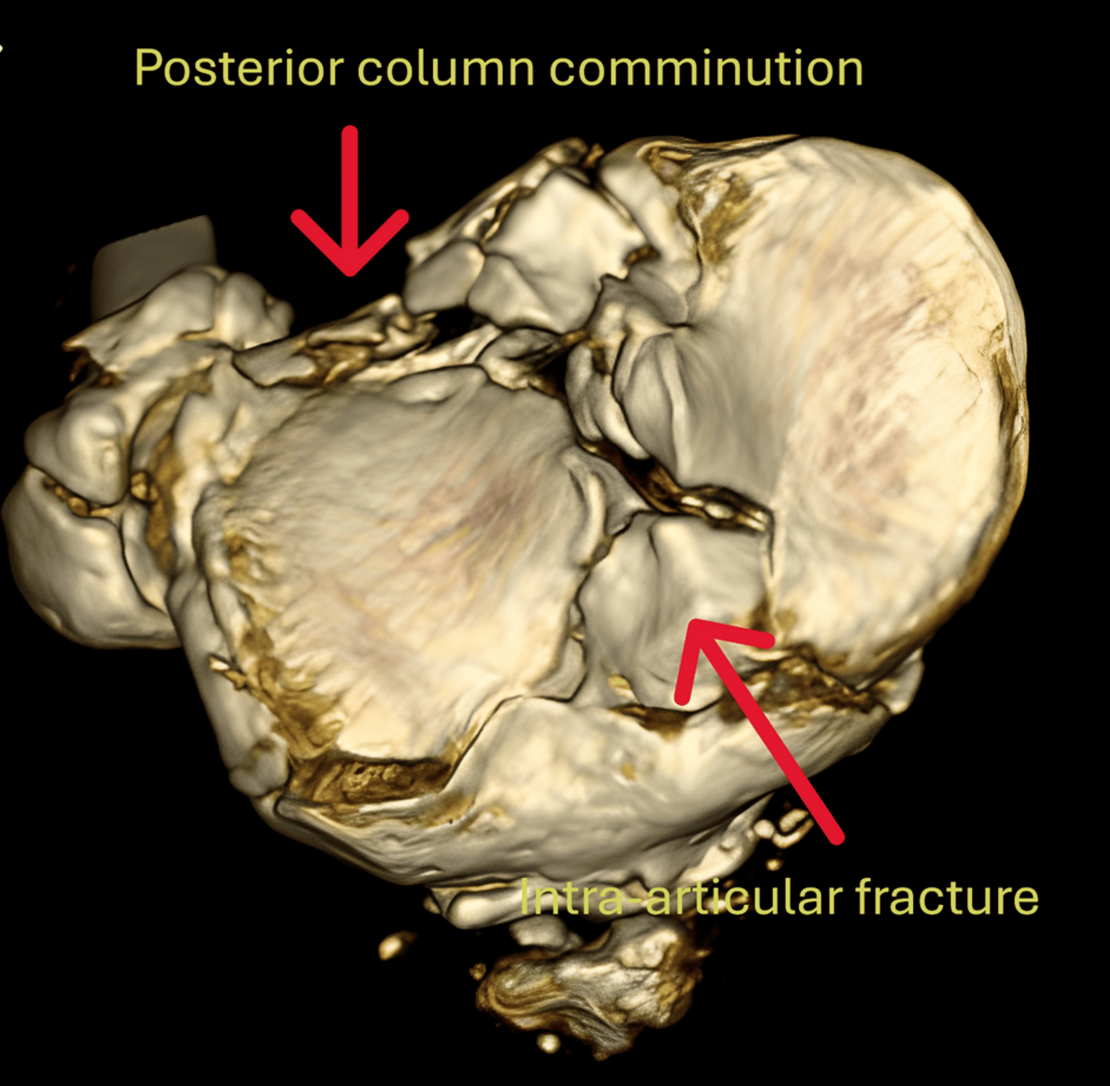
Axial 3D CT scan revealing posterior comminution with intra-articular fracture of the tibial plateau 3D CT: three-dimensional computed tomography
